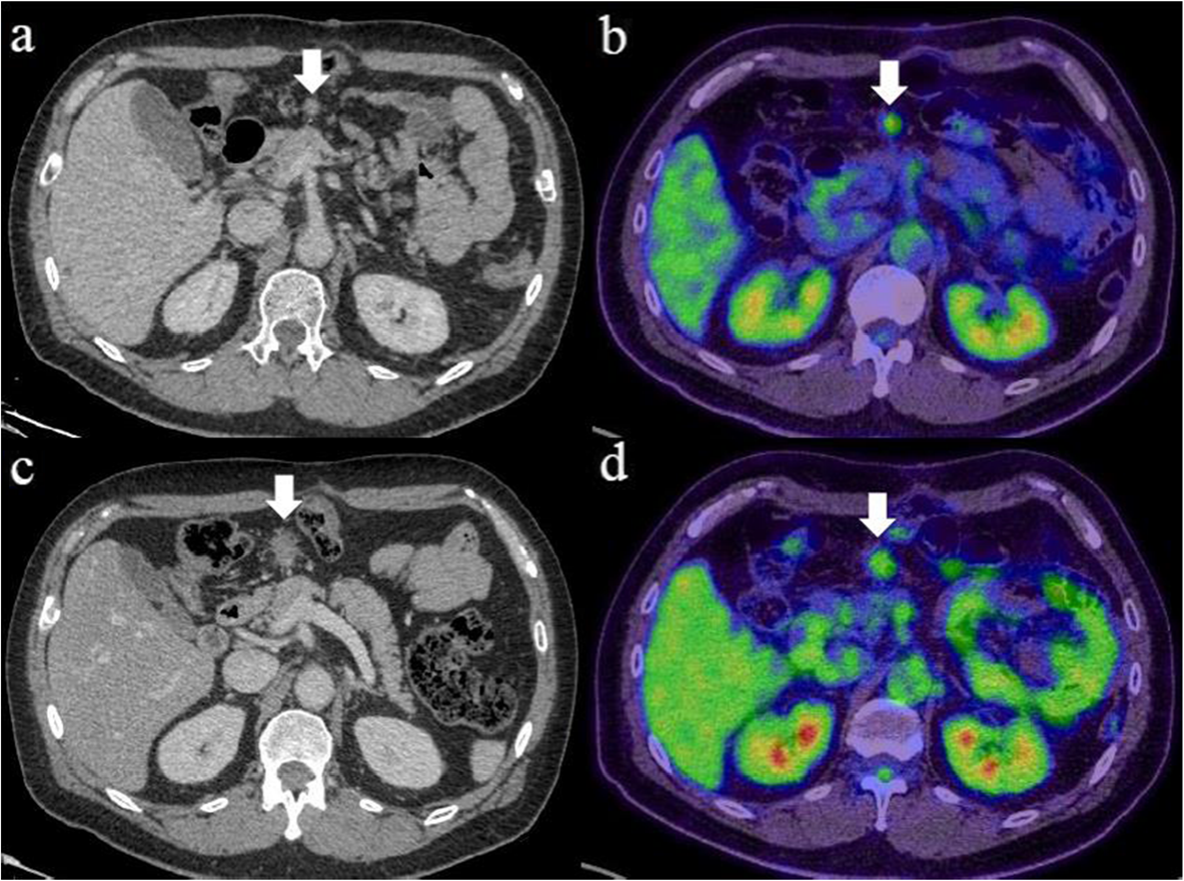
Abdominal computed tomography (CT) and positron emission tomography (PET) of the desmoid tumor. (a) CT shows a solitary and localized tumor in the mesentery of the transverse colon 27 months after gastrectomy (arrow). (b) PET shows radiotracer fluorodeoxyglucose uptake of the tumor (arrow). (c) CT and (d) PET of the tumor after five courses of chemotherapy, showing that no significant clinical response was seen (arrow).
